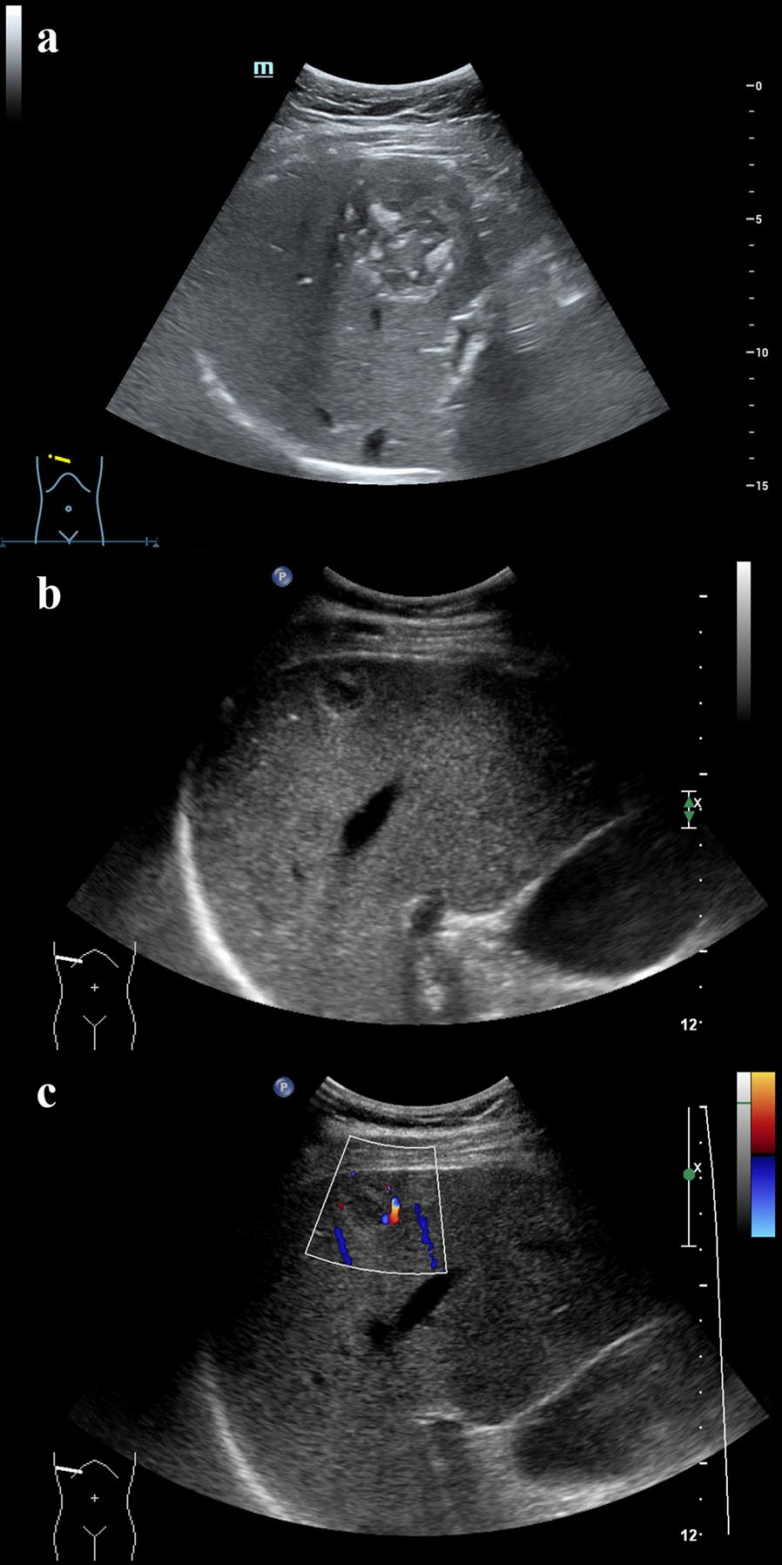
The US ultrasonogram of CE and AE. a A CE lesion exhibited circumscribed margin, regular shape and heterogeneous echogenicity with scattered calcification foci. b An AE lesion exhibited ill-defined margin, regular shape and heterogeneous echogenicity. c color Doppler ultrasonogram corresponding to b showed tiny punctiform and linear blood flow signals inside the lesion. *US* conventional ultrasound, *CE* cystic echinococcosis, *AE* alveolar echinococcosis
